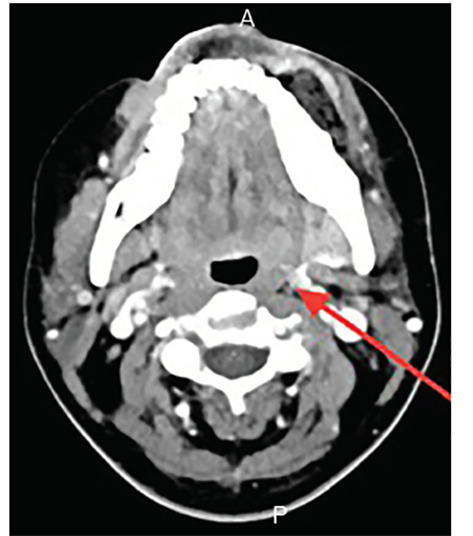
Computed tomography soft tissue neck with intravenous contrast demonstrating thickening in the left submandibular soft tissues concerning for cellulitis or the sequela of thrombophlebitis (arrow), with thrombus of the left anterior jugular vein and suspected thrombus of the superficial branches of the superficial facial vein branches and left perimandibular region.
